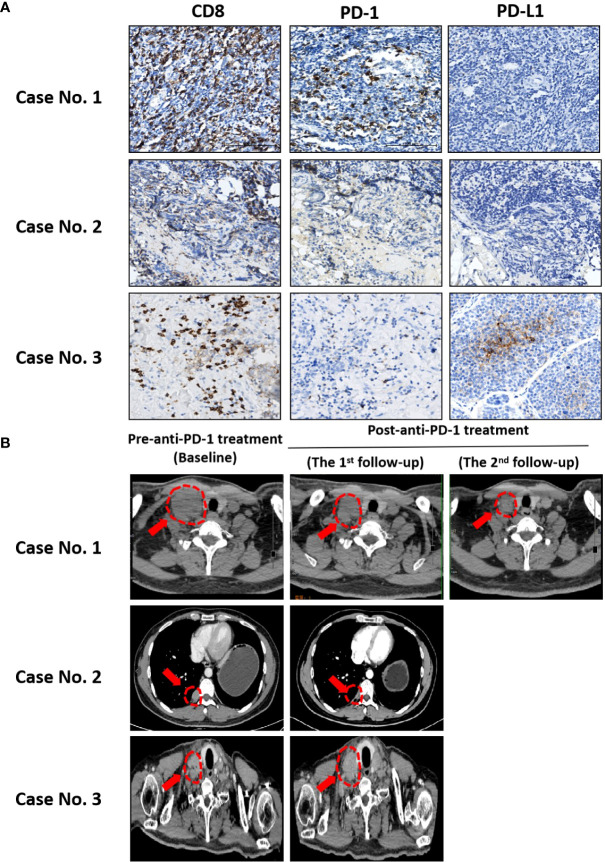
Clinical practice of ICB with toripalimab in patients with metastatic T-NEN. (A) Representative IHC images showing CD8, PD-1, and PD-L1 expression patterns in case No.1, 2, and 3. (B) CT scans at baseline (pre-anti-PD-1 treatment) and re-examinations after anti-PD-1 treatment at indicated time points are shown. The arrow points to the lesions (with red marks) that changed significantly upon toripalimab treatment.
